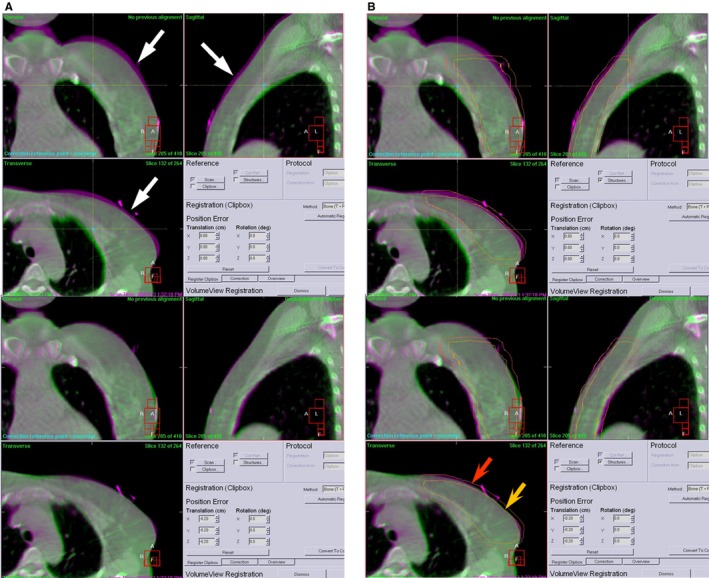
A, This breast CBCT image in upper before autofusion mapping; white arrow tip pointed out the predominant skin gap in pink color; after autofusion in lower, this skin gap could disappear. B, This breast CBCT image before autofusion mapping in upper; red arrow tip showed PTV; yellow arrow showed CTV irradiation field; after autofusion in lower, CTV can nearly matched breast CT image. CBCT, cone‐beam computed tomography; CTV, clinical target volume; PTV, planning target volume
